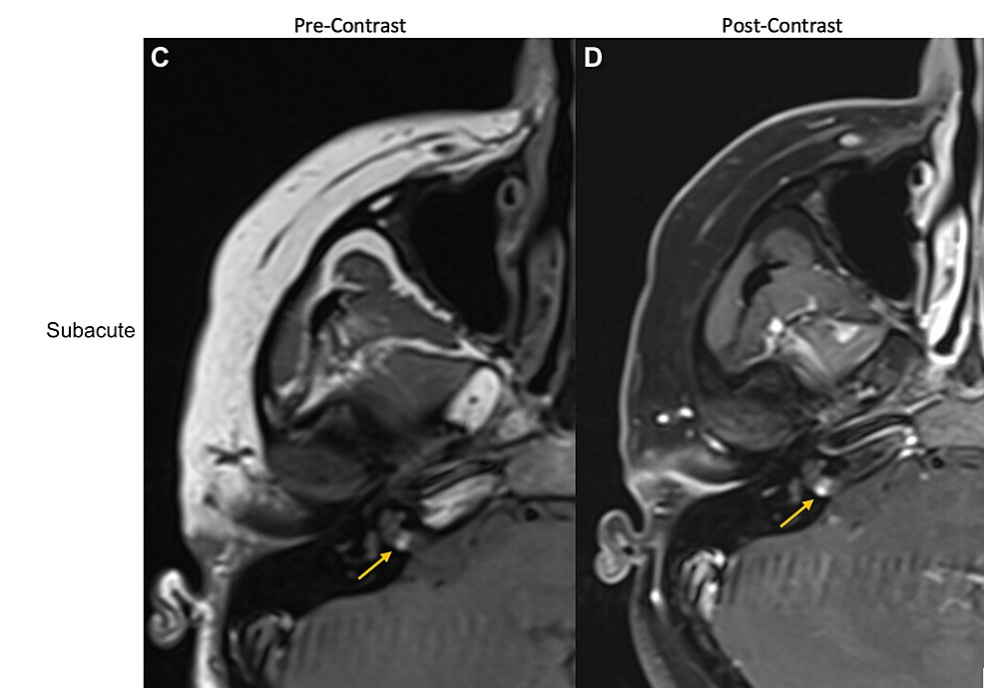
Right IAC lesion on MRI with and without contrast at subacute (two days after presentation) time point C: Pre-gadolinium T1-weighted axial MRI IAC during early subacute period showing a hyperintense right IAC lesion D: Post-gadolinium T1-weighted axial MRI IAC showing a homogeneously enhancing 3 x 5-mm soft tissue lesion in the right IAC IAC: internal auditory canal; MRI: magnetic resonance imaging
